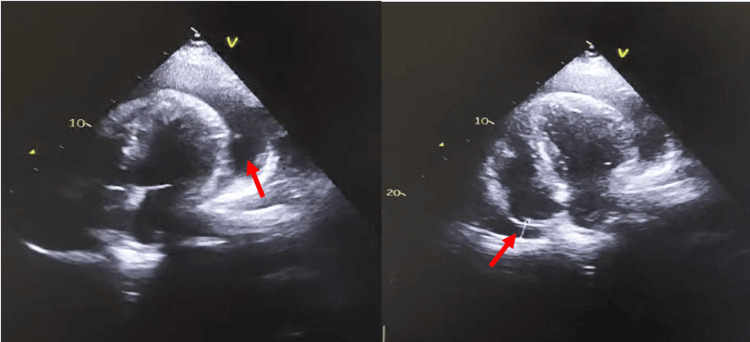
Preoperative transthoracic echocardiography showing circumferential pericardial effusion
